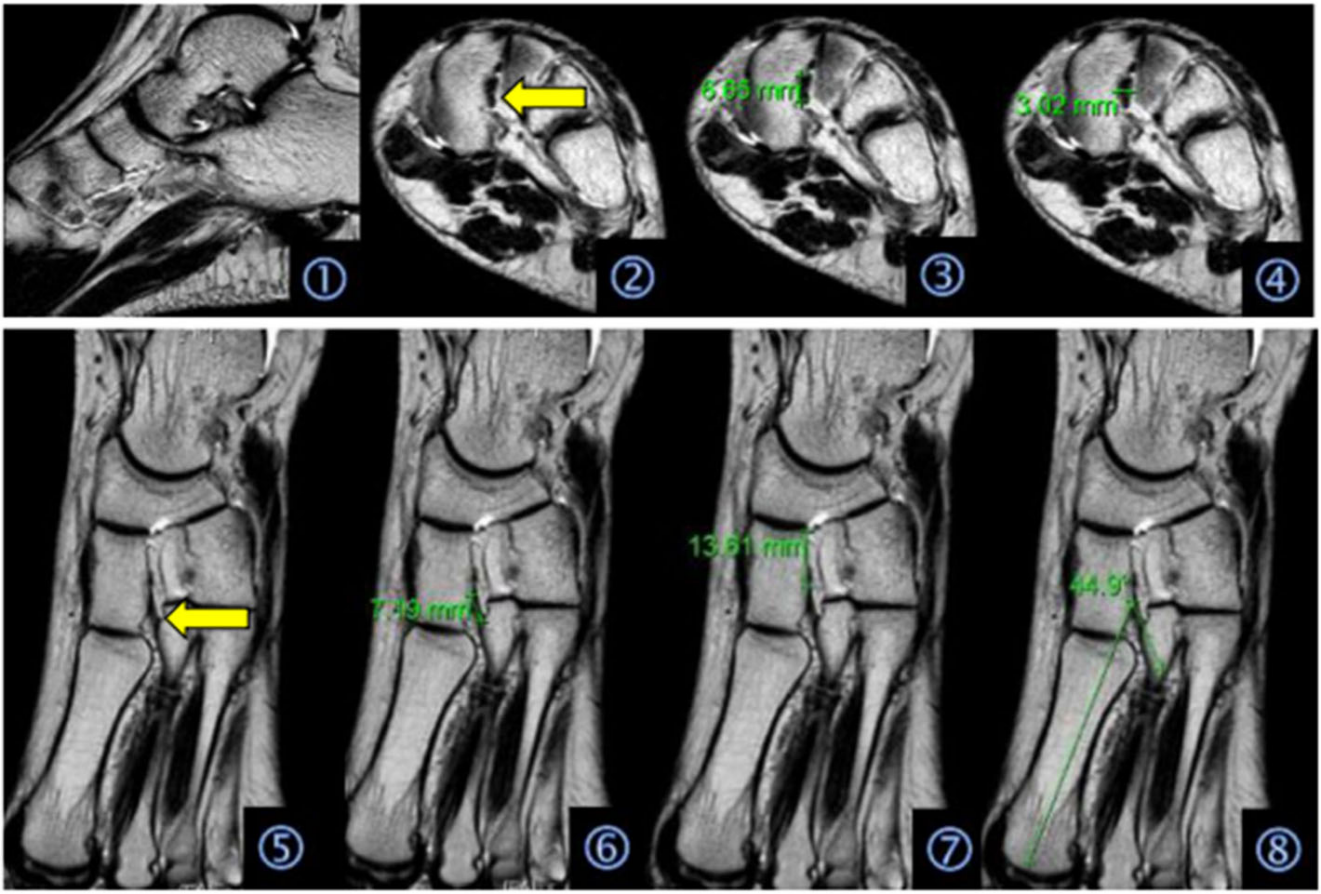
Graphic interpretations: (1) MRI scanning images of the sagittal section; (2) MRI scanning images of the oblique transverse section, where the arrow indicates the Lisfranc ligament; (3) height measurement of the Lisfranc ligament; (4) width measurement of the Lisfranc ligament; (5) MRI images of the oblique coronal section, where the arrow indicates the Lisfranc ligament; (6) length of the measurement of the Lisfranc ligament; (7) measurement of the distance between the origin of the Lisfranc ligament and the base of the medial cuneiform bone; and (8) measurement of the included angle between the Lisfranc ligament and the long axis of the first metatarsal bone
